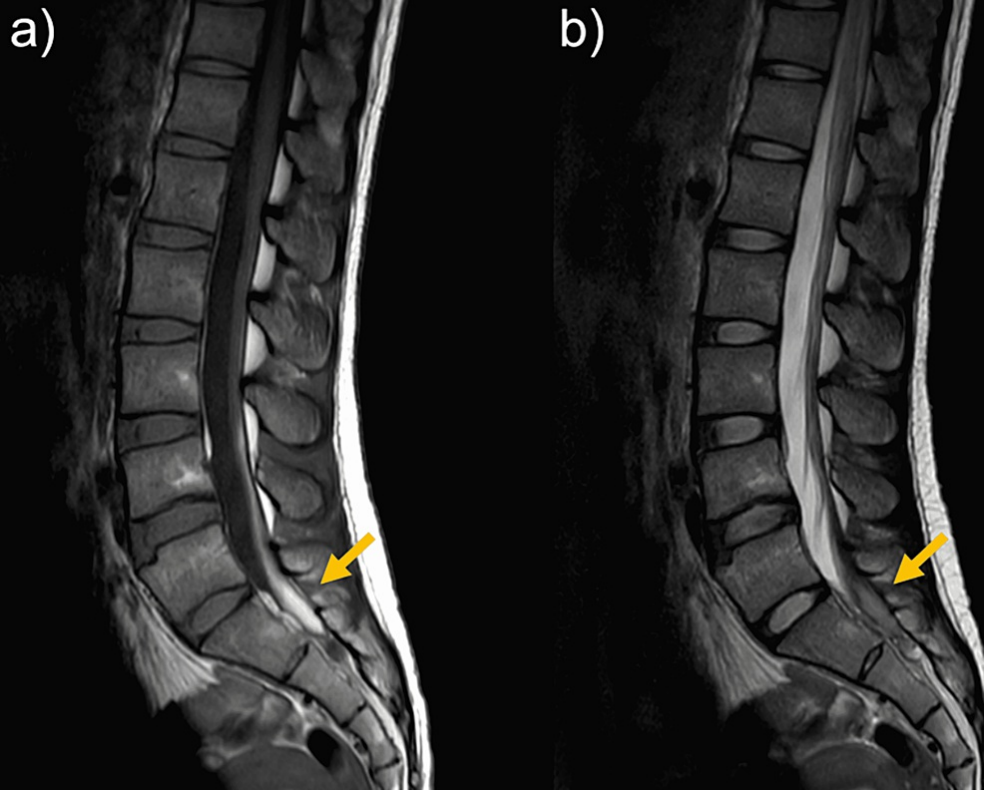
Lumber MRI at seven days after left leg pain appeared (a) Diffuse hematoma-like mass with a high signal on T1-weighted imaging. (b) An isointense signal on T2-weighted imaging
